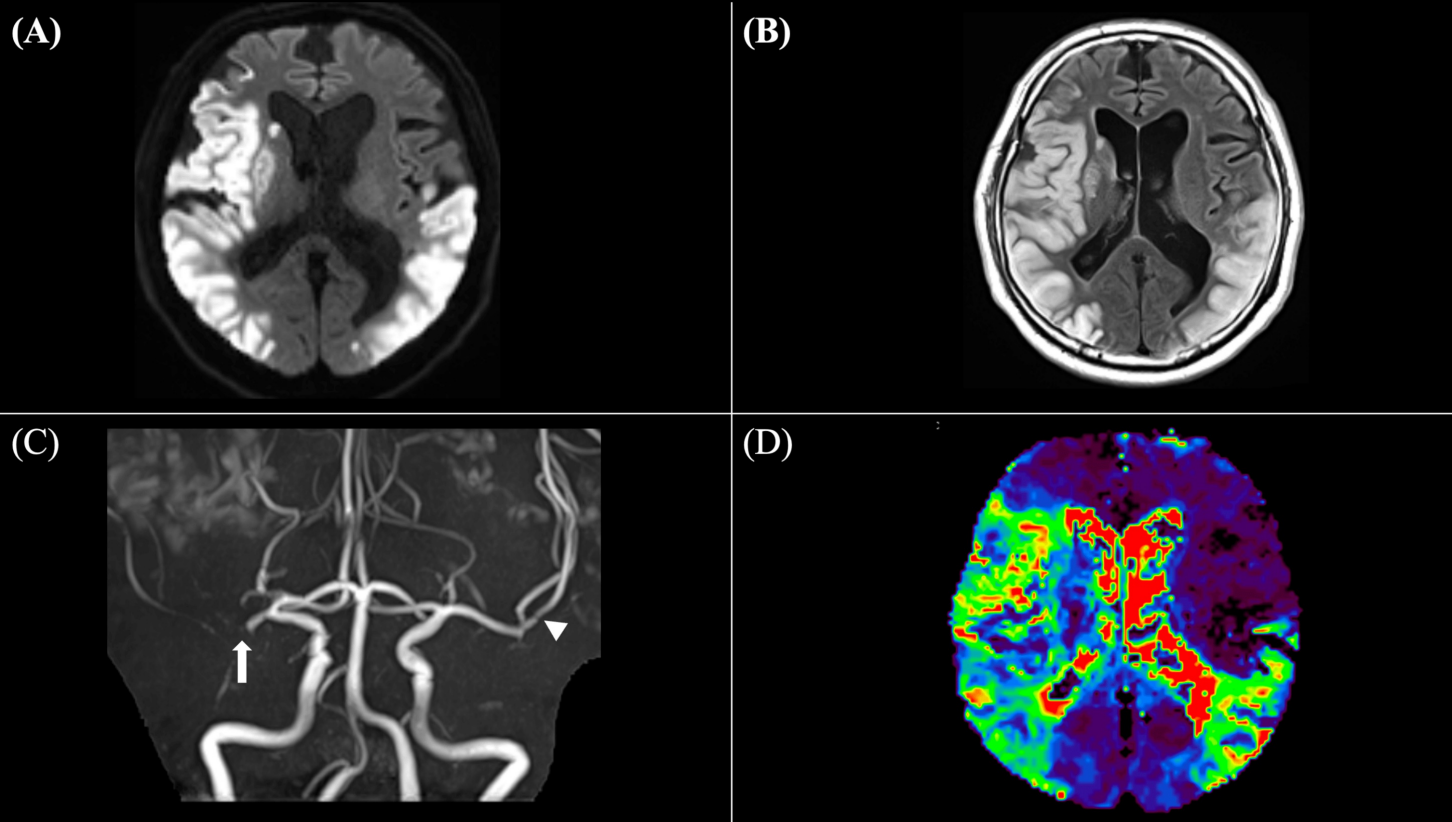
Follow-up MRI findings on hospital day 2 A: diffusion-weighted imaging (DWI) shows no significant enlargement of the infarcted area, suggesting no progression into the subcortical or deep white matter; B: fluid-attenuated inversion recovery (FLAIR) imaging reveals worsening cytotoxic and vasogenic edema in the cortical infarction regions; C: time-of-flight (TOF) magnetic resonance angiography (MRA) demonstrates persistent right middle cerebral artery (MCA) occlusion (arrow) and continued stenosis of the left MCA (arrowhead), without evidence of recanalization; D: perfusion-weighted imaging (PWI) using time-to-max (Tmax) maps shows no significant change in perfusion deficits compared to the initial MRI, indicating persistent cerebral hypoperfusion.
